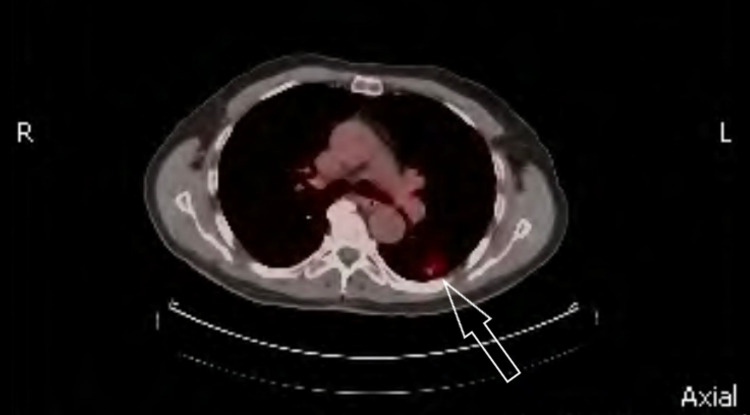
A PET/CT scan demonstrating a nodule in the posterior aspect of the left lower lobe of the lung
